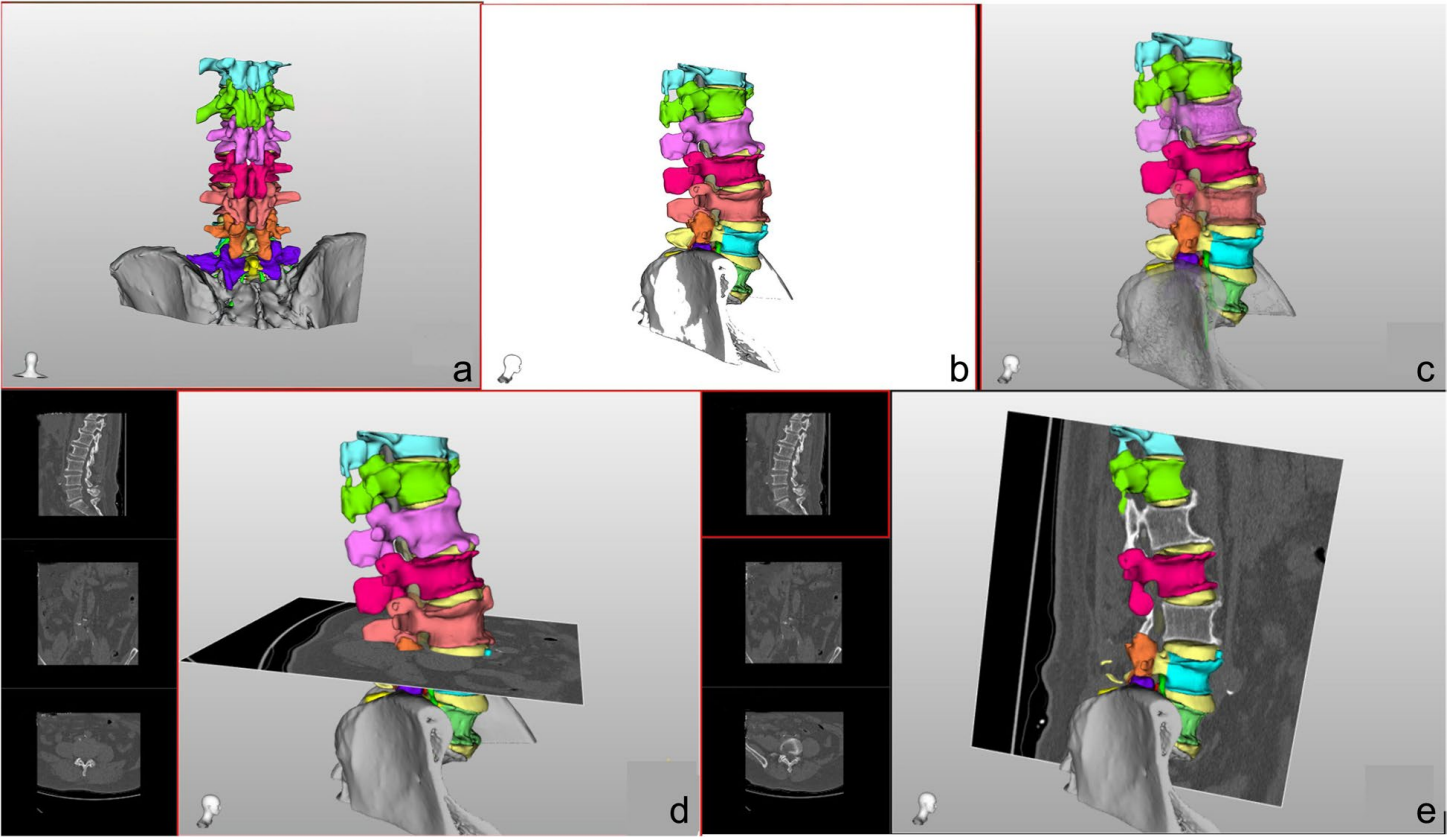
a Back view and b Side view. c The L1, L3 and pelvis of the model were transparent. d Combining the CT cross-sectional image with the model, you can slide up and down to adjust different CT planes. e CT sagittal image combining the model and processes L1 and L3 with hidden instructions
